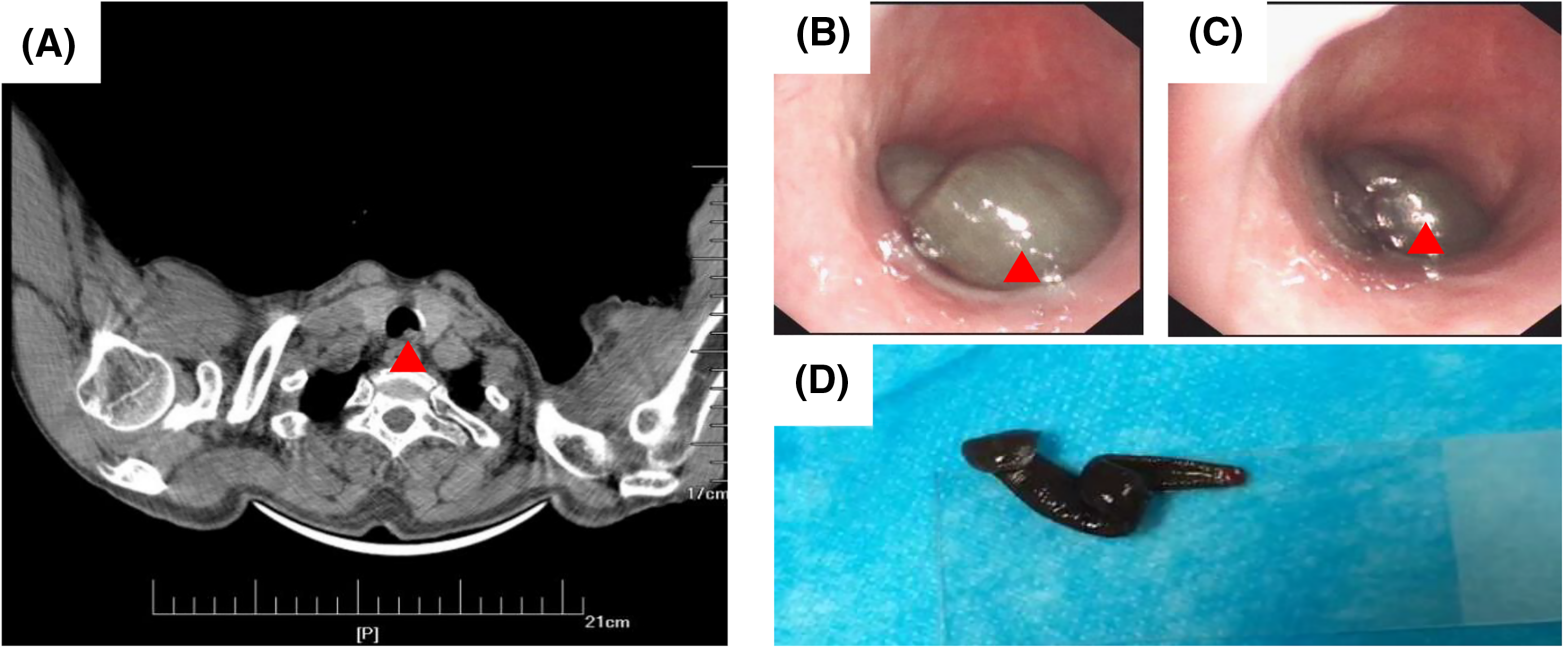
(A) Computed Tomography (CT) showing soft tissue shadow in the upper trachea. (B,C) The leech in the upper trachea. (D) The leech was removed from the patient.
